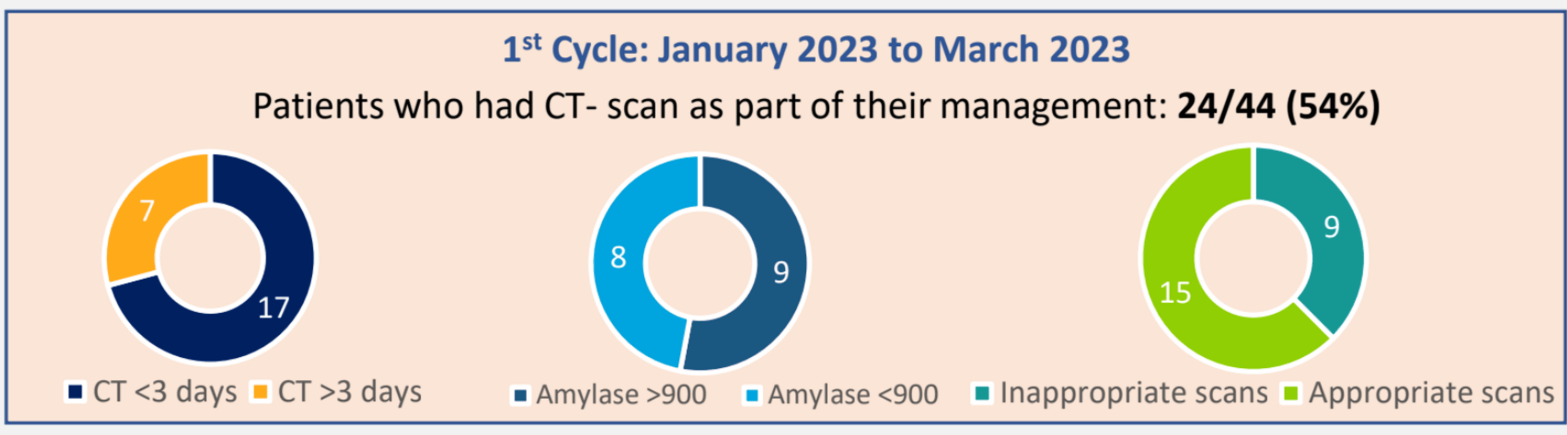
Results of the first cycle of the audit CT: computed tomography
